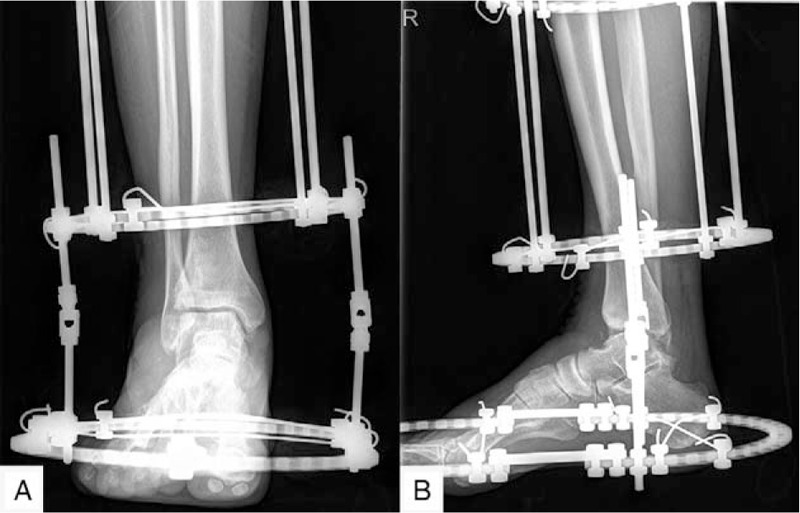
The radiograph 1st week postoperative showed good joint space and alignment.
